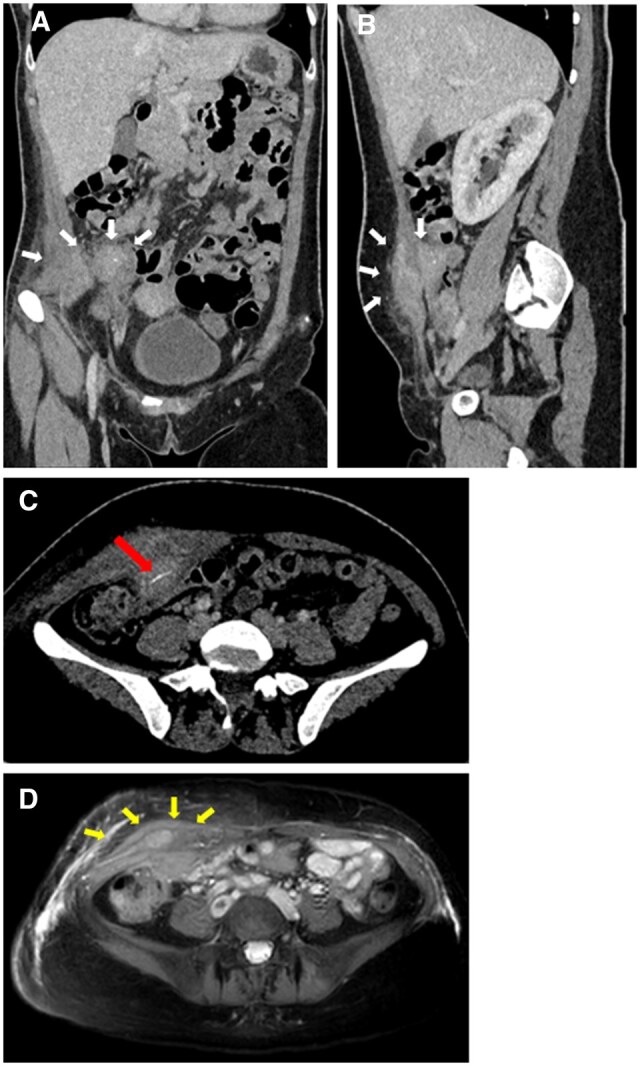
Case 2. Coronal (A), sagittal (B) and axial (C) CT with PV contrast as well as axial MRI T2 fat saturated (D) images showing the abdominal wall phlegmon (white arrows) and a linear hyperdense fish bone within (red arrow). The phlegmon displays a high signal on MRI T2FS images indicating edema with pockets of fluid along the right anterior subcutaneous tissues.
